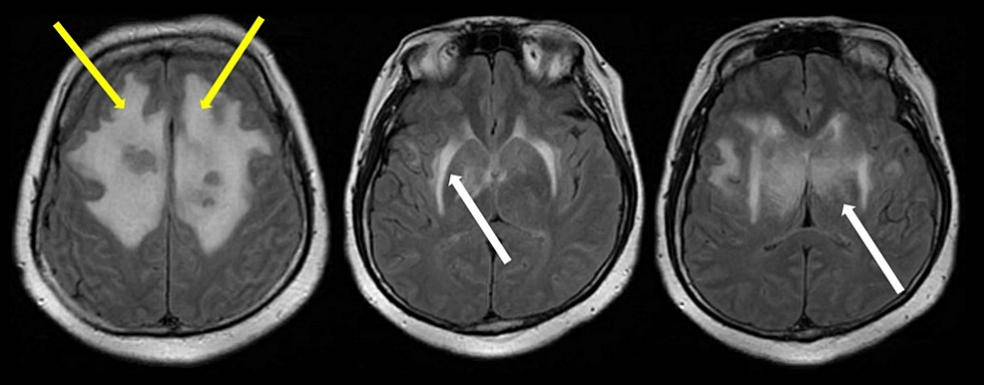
T2 FLAIR images. There is significant vasogenic edema involving the bilateral frontal lobes (yellow arrows) as well as the basal ganglia (white arrows). FLAIR: fluid attenuated inversion recovery
